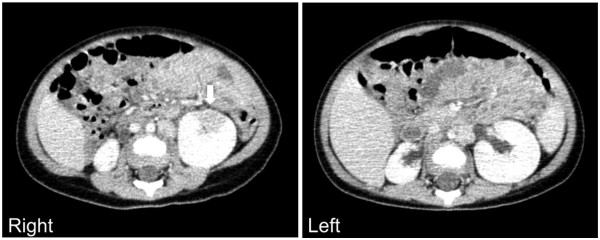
Enhanced computed tomography findings indicating left pyelonephritis. The right side image reveals decreased contrast enhancement in a part of the left renal parenchyma (arrow), indicating pyelonephritis. The left side image indicates the right small kidney and compensatory hypertrophic left kidney.
